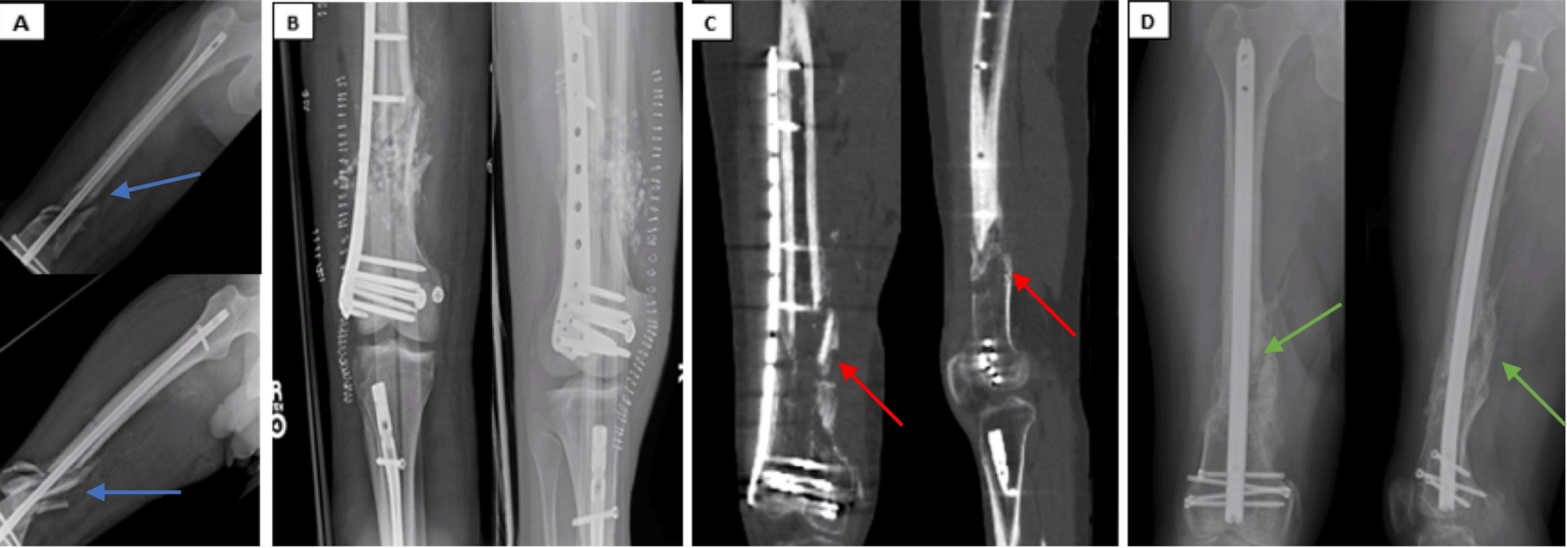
A 32-year-old man with an open, comminuted distal femur fracture that developed into an oligotrophic nonunion (case 4) A) Radiographs four weeks status post retrograde nailing for the comminuted distal third femur fracture (blue arrows) at an outside institution, with approximately two centimeters of shortening and no evidence of callus formation at the fracture site. Ipsilateral tibial nailing was performed at the time of initial retrograde femoral nailing for an open tibia fracture. B) Immediate postoperative imaging status post femoral nail removal with revision to lateral distal femur locking plate and bone grafting. C) CT scan eight months after femoral plating and bone grafting operation demonstrating a persistent nonunion (red arrows). D) Radiographs nine months after exchange nailing with pre-drilled cortical vent tunnels demonstrating robust bridging bone formation (green arrows) at the former nonunion site.
